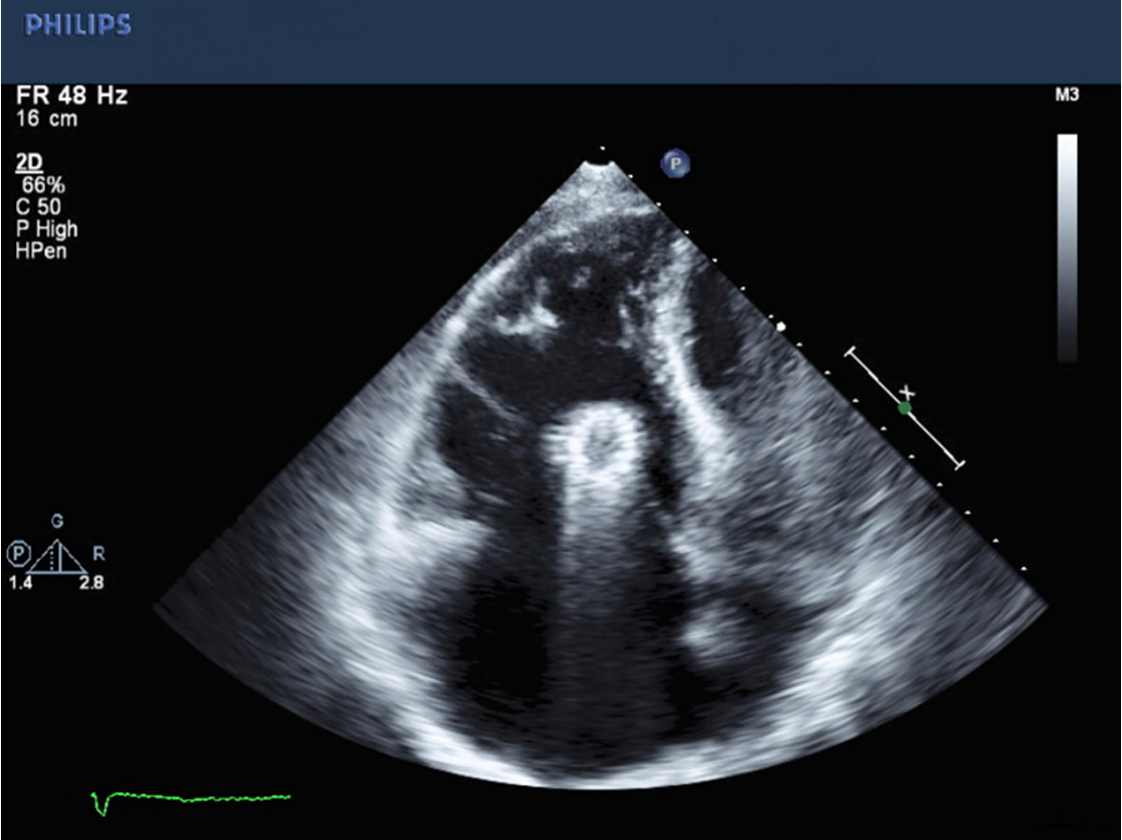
Modified apical four‐chamber view showing entrapment of the stent within the tricuspid valve.
